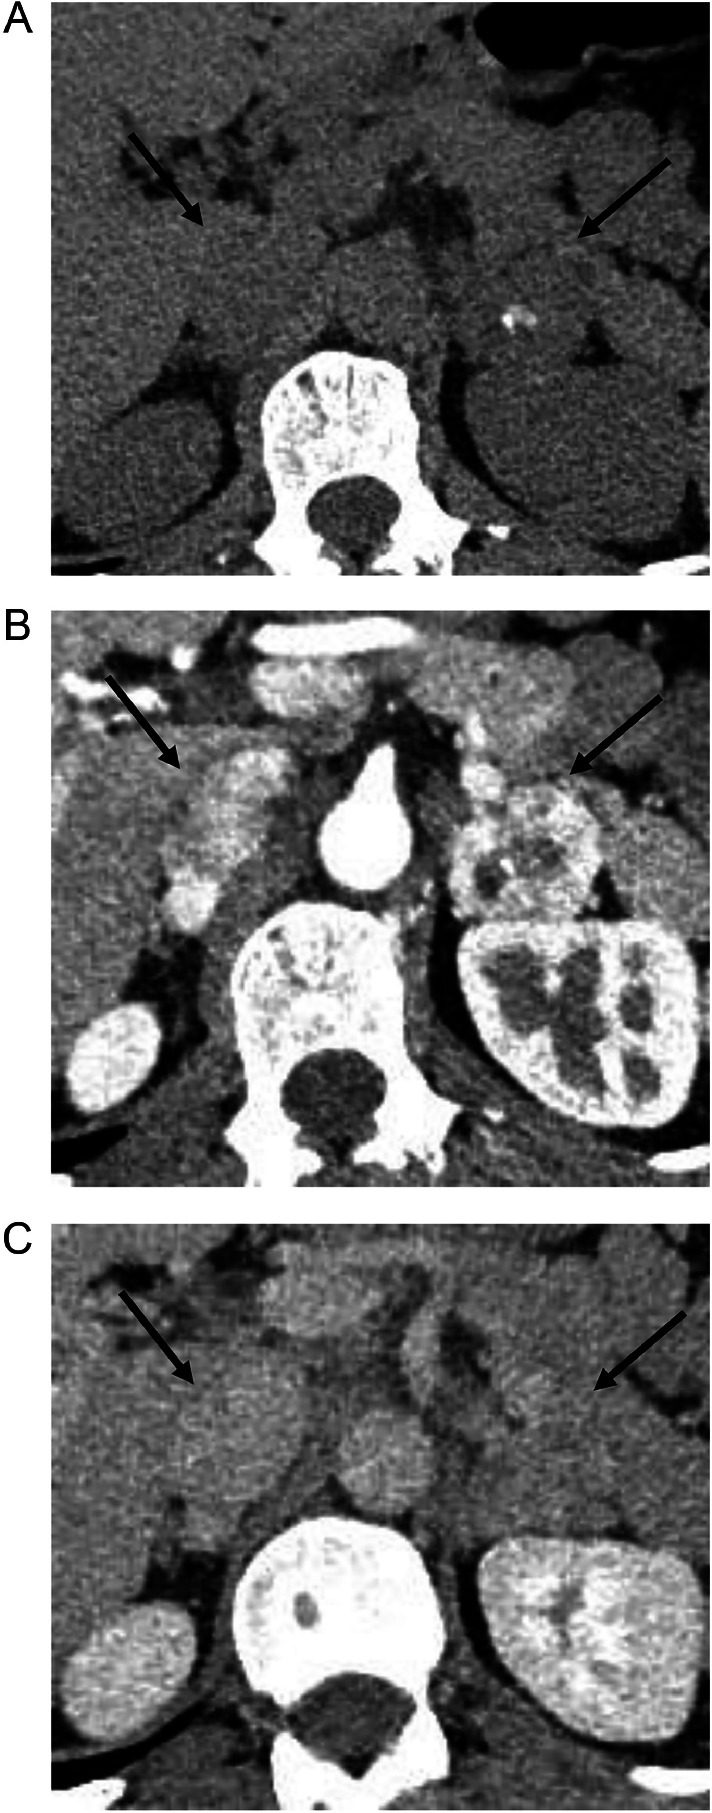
Dynamic CT images of the adrenal glands. Dynamic CT axial images show hyper-vascular masses in bilateral adrenal glands (black arrows) (A-C). Calcification is noted within the left adrenal solid mass (A). a) Pre-contrast phase; B) early phase; C) delayed phase.
